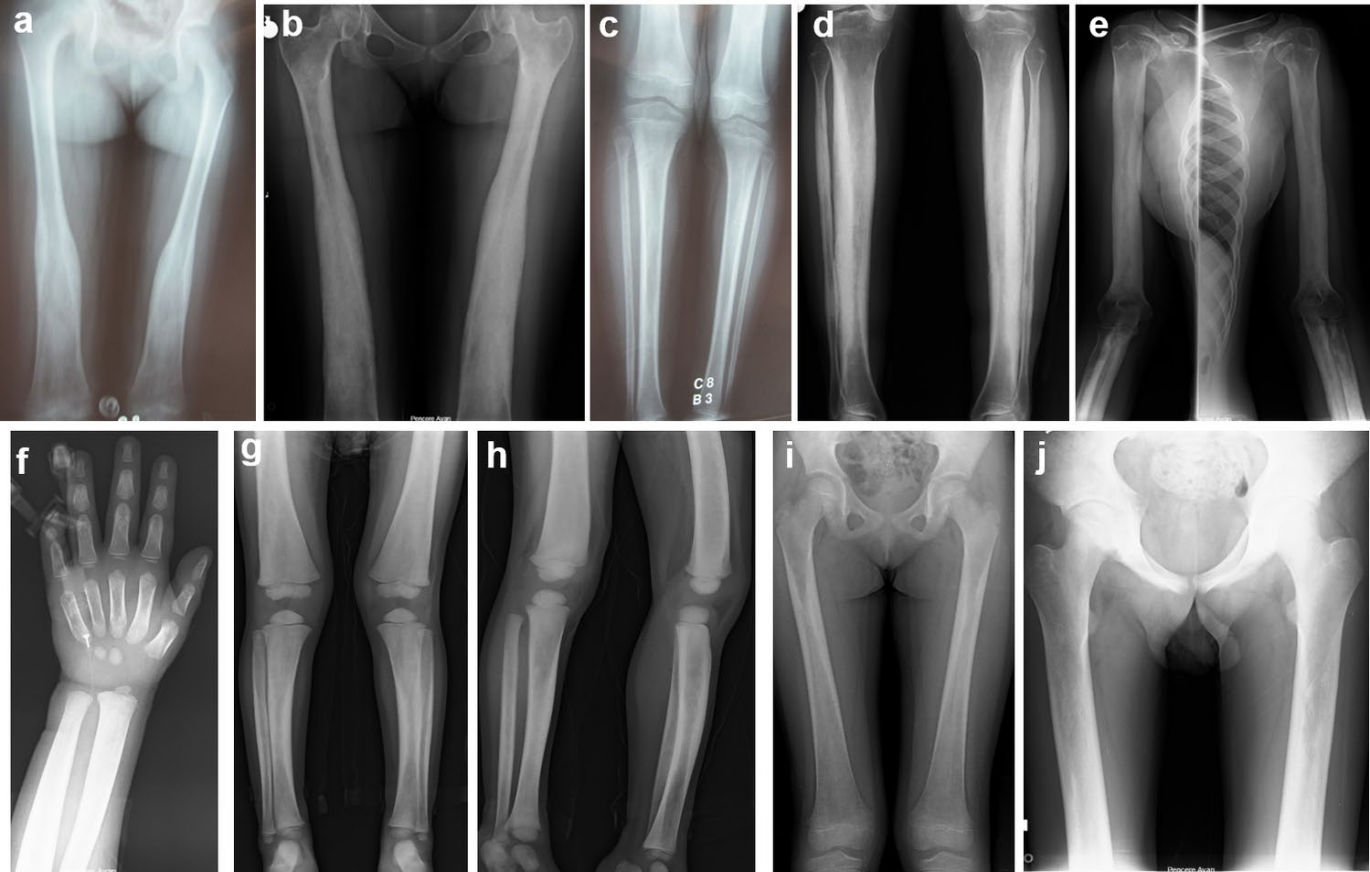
Patient 7 with Camurati–Engelmann disease at 9.5 (a, c) and 17 years (b, d, e). Note progressive diaphyseal involvement, periosteal hyperostosis, cortical thickening, and Erlermeyer flask deformity. Patient 12 at the age 3 (f–h) and her sister (P13) at 6.5 (i) and P11 at 18 years with Ghosal hematodiaphyseal dysplasia (j). Radiographs revealed diaphyseal widening and sclerosis, and cortical thickening
